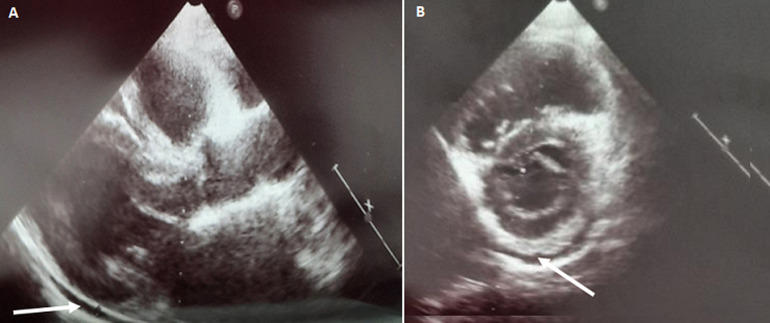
(A,B) pericardial effusion, in the cardiac ultrasound, in an adult patient with thiamine-responsive megaloblastic anaemia (TRMA) on discontinuation of oral thiamine-based treatment
